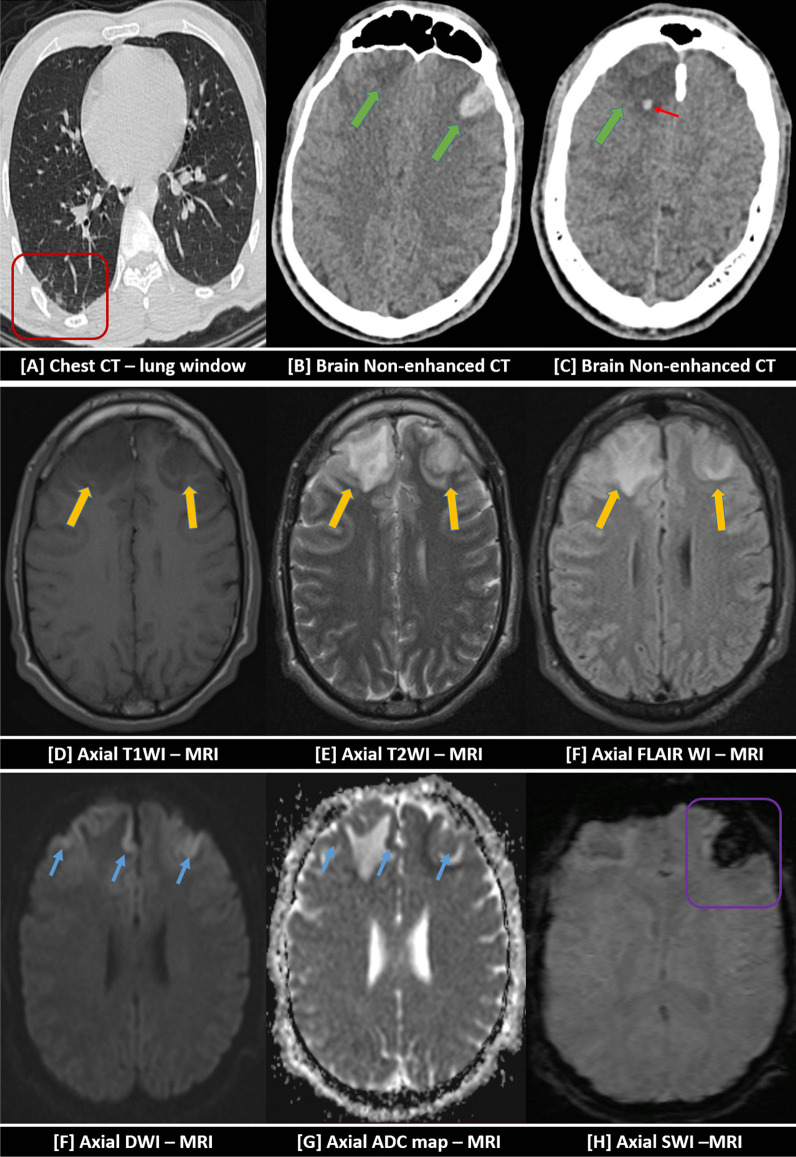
An 84-years-old female patient was proved for COVID-19 infection after one week of cough and mild fever. She presented to the emergency unit with a suddenly disturbed level of consciousness. [A] Chest CT examination (lung window) revealed small right basal sub-pleural ground-glass patches of COVID-19 infection (red square). [B, C] Urgent non-contrast brain CT revealed bilateral high frontoparietal cortical and sub-cortical heterogeneous mixed hypo and hyper-dense areas (green and red arrows) … The initial diagnosis was bilateral hemorrhagic infarctions. An initial non-contrast MRI examination was performed. They express heterogeneous hypointense signals in T1-WI [D] and mixed iso and hyperintense signals in T2-WI [E] and FLAIR-WI [F] (yellow arrows). Positive diffusion restriction was noted with cortical bright DWI signal [G] and low ADC value in ADC mapping [G] (blue arrows). Hemorrhagic transformation was evidenced by positive SWI signal blooming [H] (violet square) … Hyper-acute hemorrhagic transformation was diagnosed
